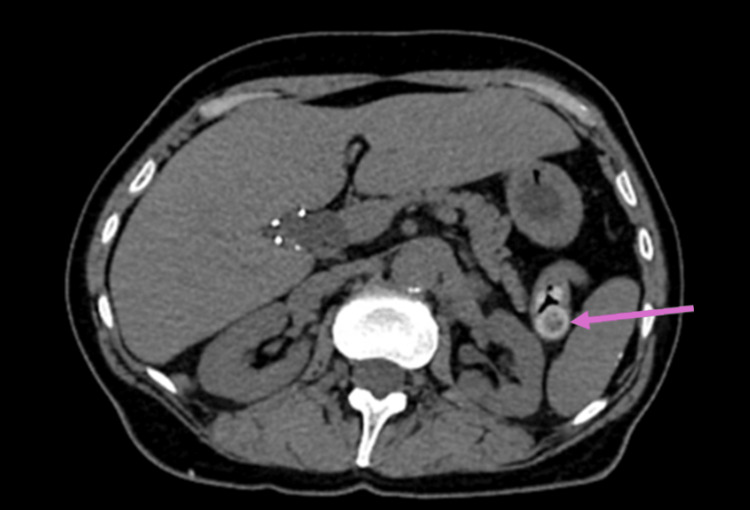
Incidental small bowel mass seen on CT angiography (pink arrow) CT: computed tomography
